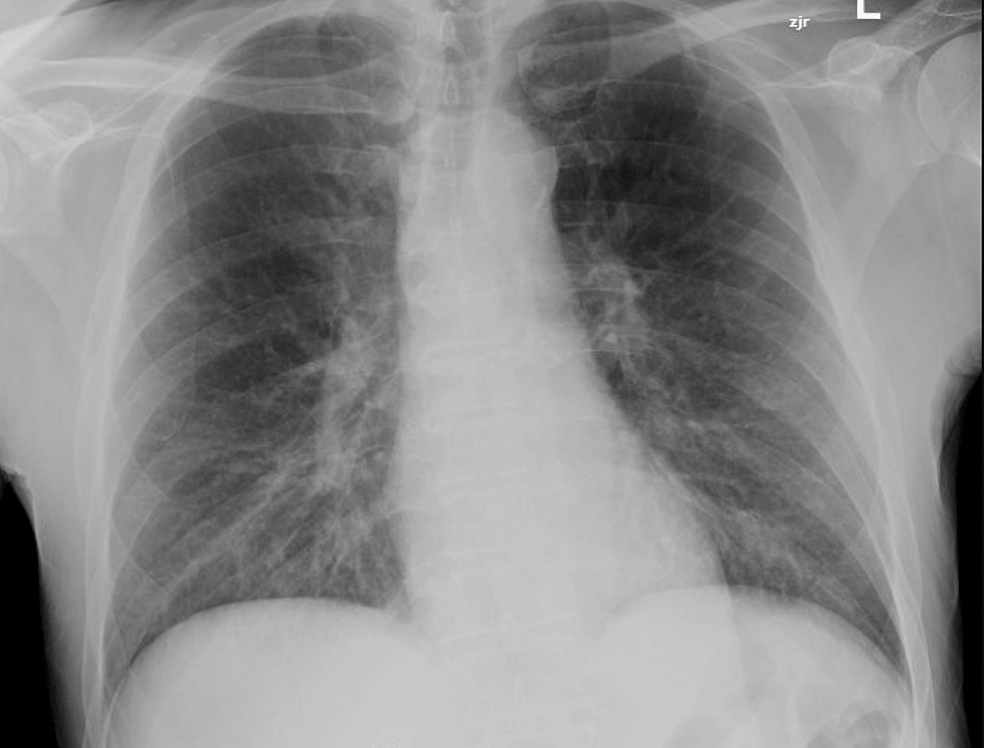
Initial chest radiograph: normal chest radiograph
